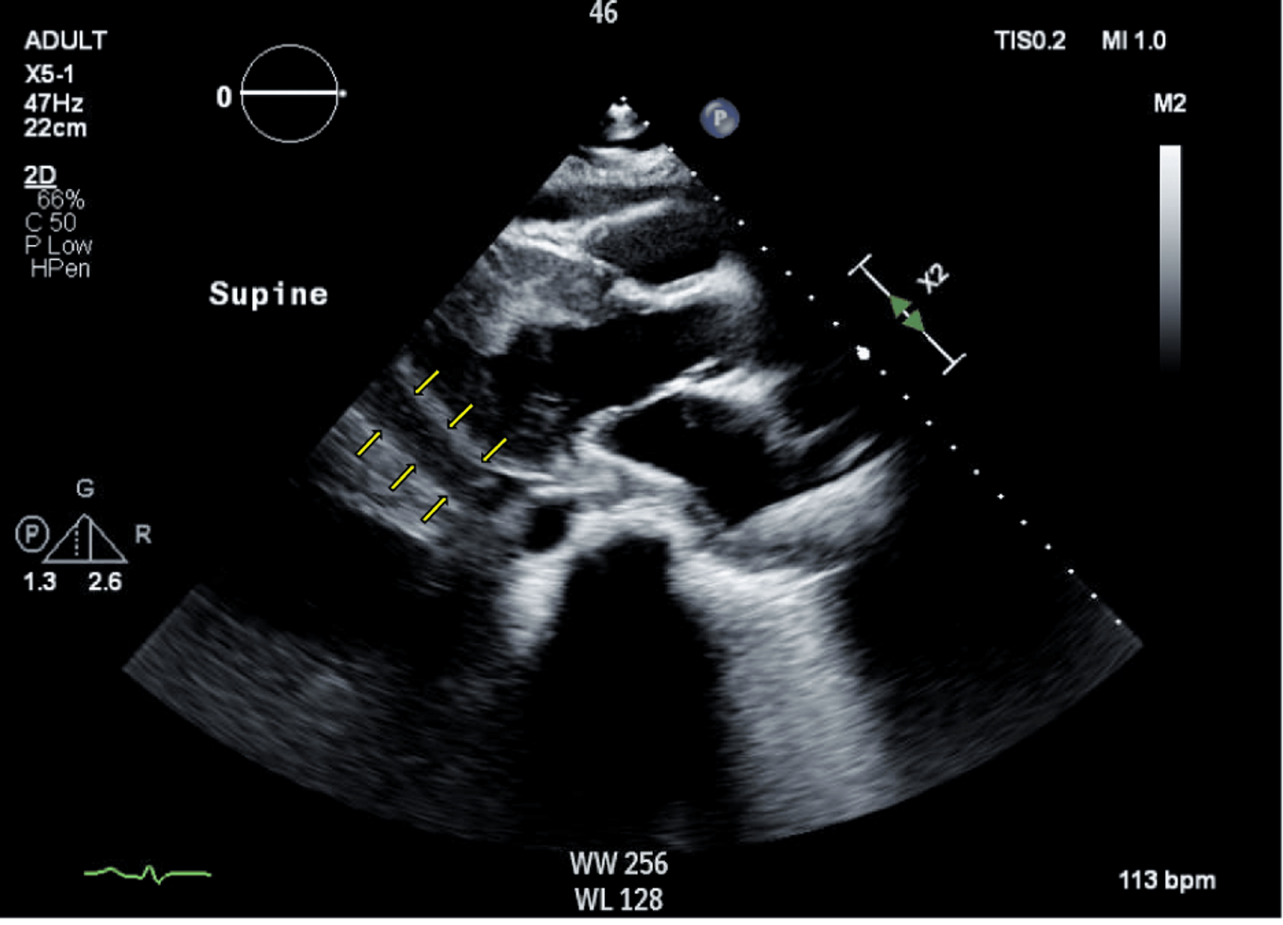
Echocardiogram showing pericardial effusion Echocardiogram showing pericardial effusion (yellow arrow). Fluid collection was followed throughout the hospital stay to determine resolution of the pericardial effusion.
